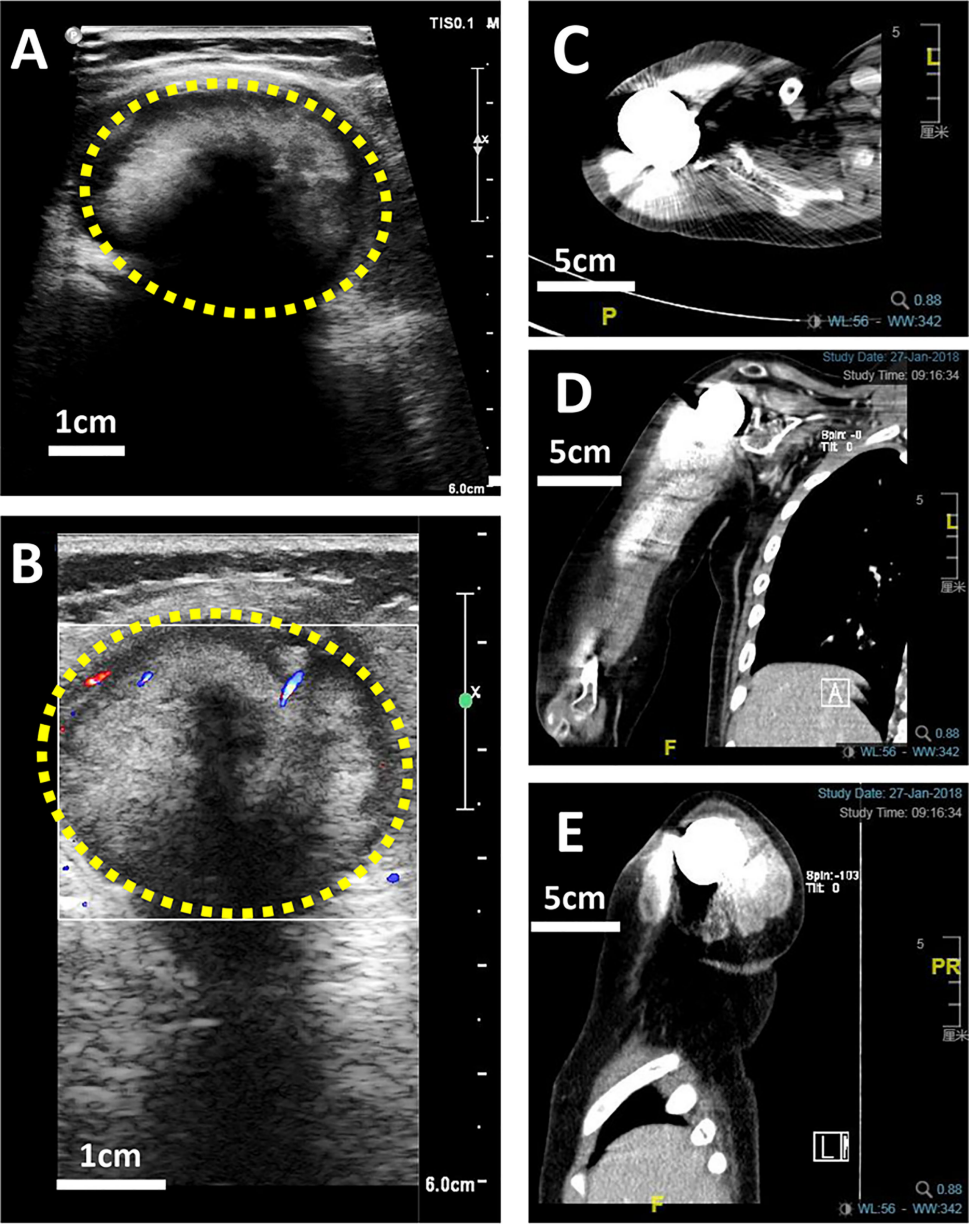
US and CT images of a 10-year-old boy with OS local recurrence after limb salvage with metal implants. It was easy to see the lesion in US but not in CT due to the imaging artifacts of metallic prosthesis. (A) A heterogeneous intramuscular hypoechoic mass in the right shoulder in US image. (B) Color Doppler US image of the mass. (C) The transverse plan of CT image. (D) The coronal plan of CT image. (E) The Longitudinal plan of CT image.
